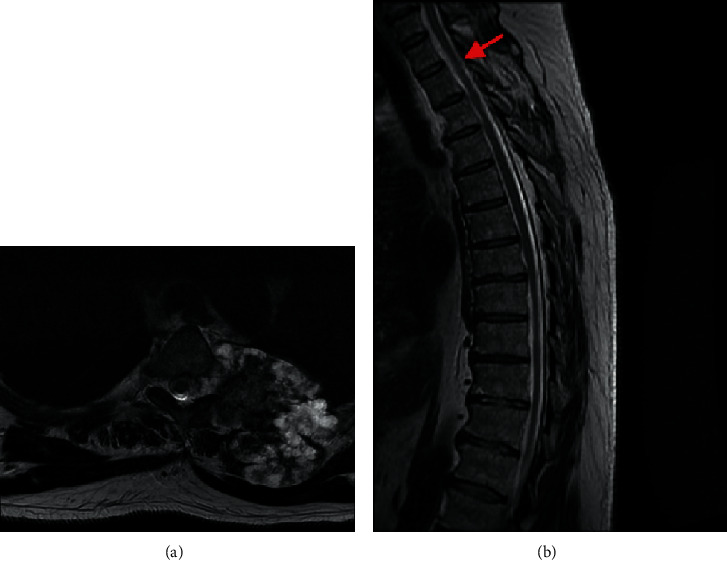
T2 MRI of patient's thoracic spine: (a) axial view at T4 level; (b) midline sagittal with arrow at T4.
